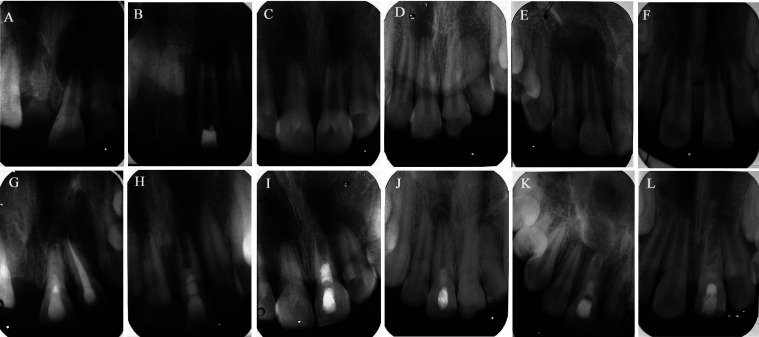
Pre-operative radiographs with periapical lesion and immature apex (A,B and C for A-PRF and D,E and F for PRF) and final follow-up respective radiographs (G,H and I for A-PRF and J, K and L for PRF). (G) #21, A-PRF treated, 20-year-old male with a 16-month follow-up shows periapical healing with increased root thickening and root maturation. (H) #21, A-PRF treated, 17-year-old male with 17-months follow-up shows periapical healing with no significant continuation of root development. (I) #21, A-PRF treated, 12-year-old male, a 15-months follow-up reveals periapical healing with closed root apex (J) #11, PRF treated, 12-years-old male with 12 months follow-up, minimal periapical healing with thickening of canal walls and continued root maturation (K) #11, PRF treated, 17-year-old, male with 12-months follow-up, periapical healing and root apex closed (L) #21, PRF-treated, 8-year-old female, 12 months follow-up, periapical healing with root apex closed.
